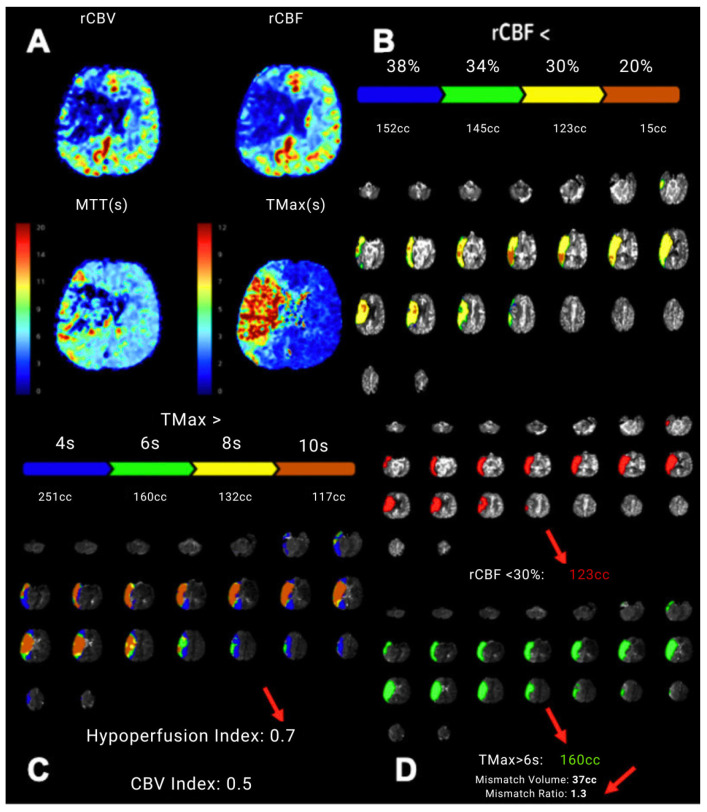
Patient case example: Evaluation of multimodal CT imaging. In the same patient: (A) CTP imaging revealed a large penumbra in the right MCA territory, involving the lateral portions of the frontal, parietal, and temporal lobes. Within this area, the core was identified in the right frontal and parietal lobes, the insula, and the right caudate nucleus. (B–D): Automated measurements were obtained using the AI-based software Viz CTP, which calculated the core and penumbra volumes, mismatch volume, mismatch ratio, and the HIR value (indicated by arrows).
